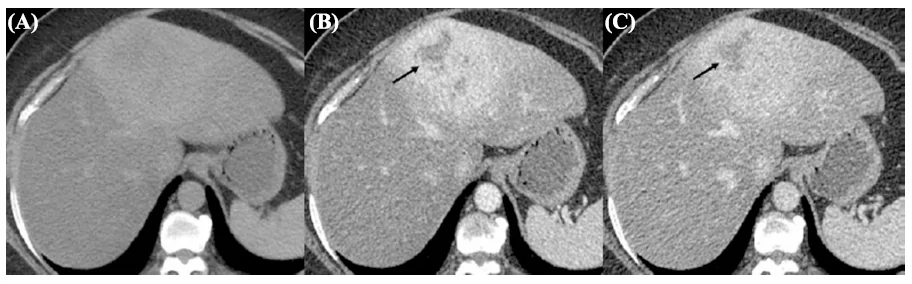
Plain and contrast-enhanced CT images.
